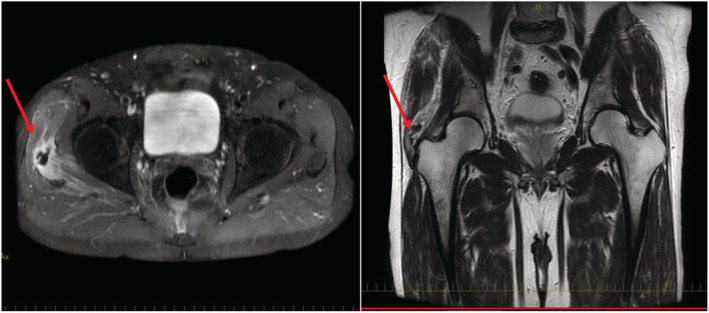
Magnetic resonance imaging revealed low‐intensity proximal to the greater tuberosity of the right femur on T1 and T2, and high intensity of muscles around the calcification on STIR and contrast enhancement.
